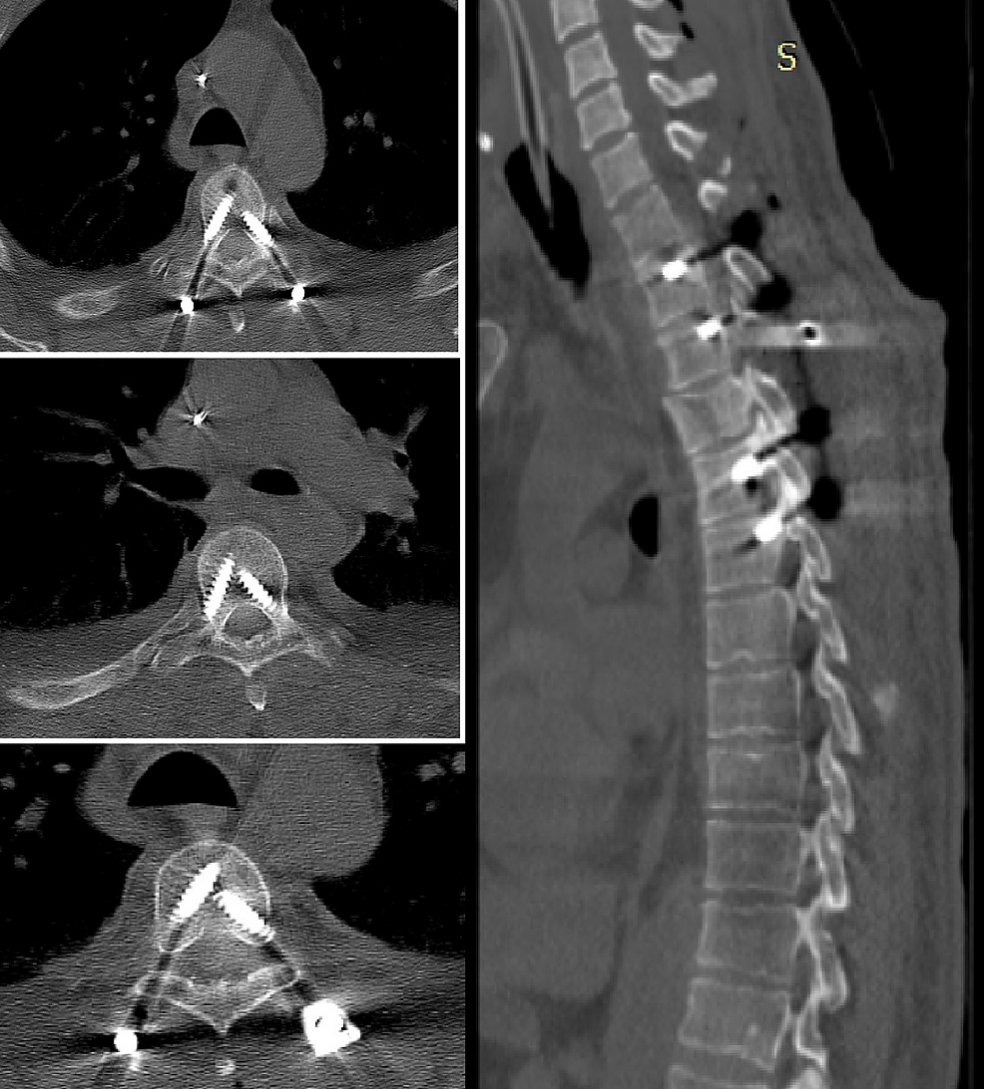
Postoperative CT image of a female patient operated with 3D modeling technique.
